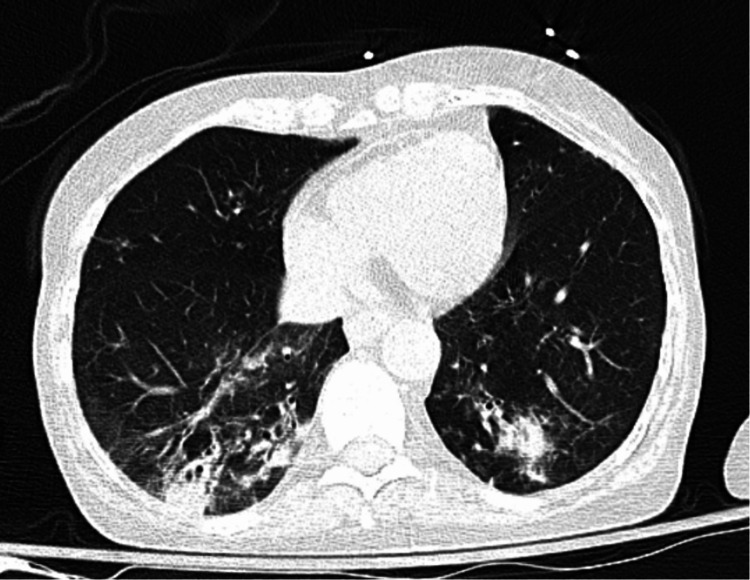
CT scan of the chest with bilateral airspace disease
